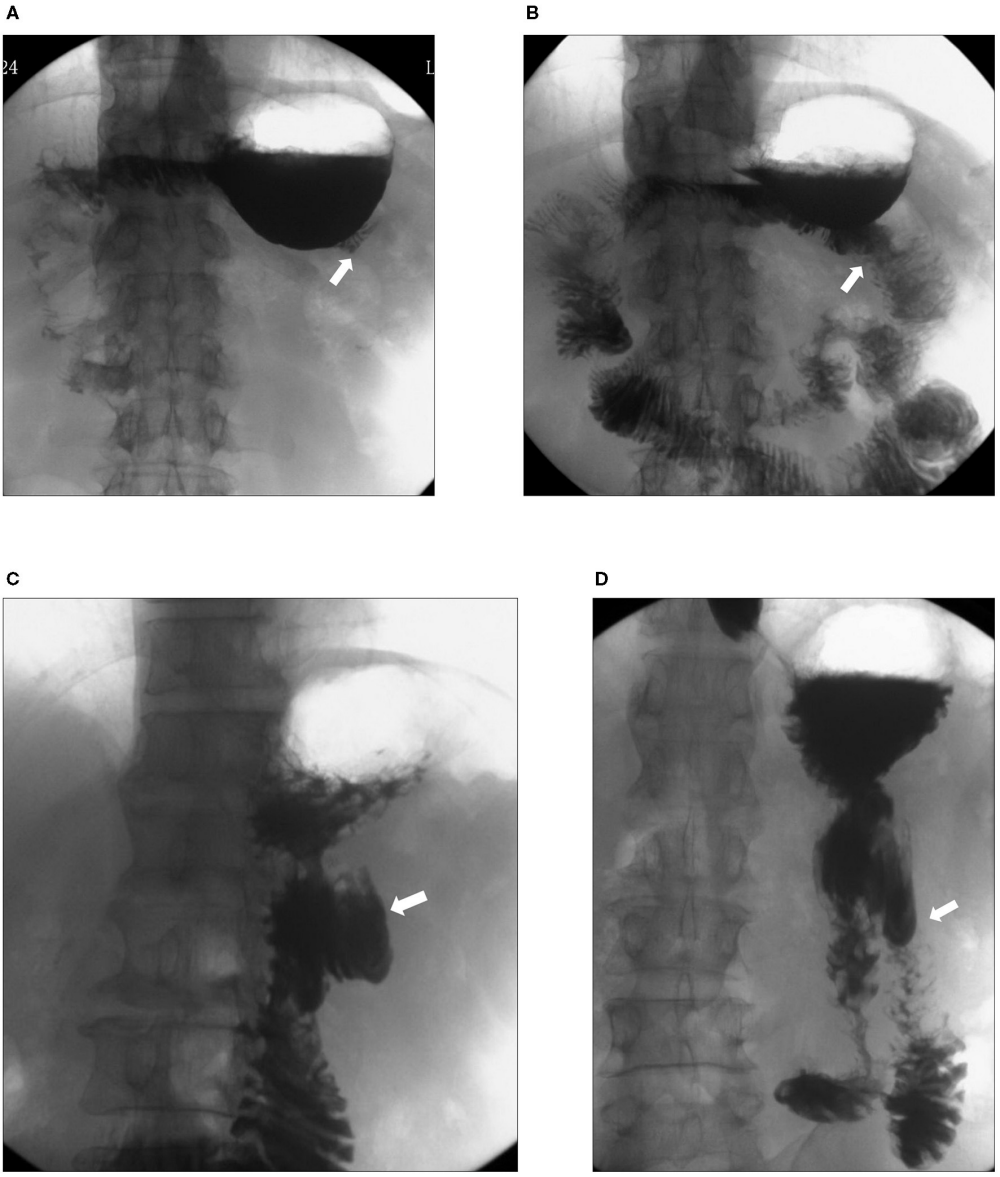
Upper gastrointestinal radiography of patients with recanalization. Upper gastrointestinal radiography showed closure of the afferent limb (white arrows) 1 month after the operation (A) and recanalization (white arrow) 3 months after the operation (B). In another patient, upper gastrointestinal radiography showed closure of the afferent limb (white arrow) 3 months (C) after the operation and recanalization (white arrow) 6 months after the operation (D).
